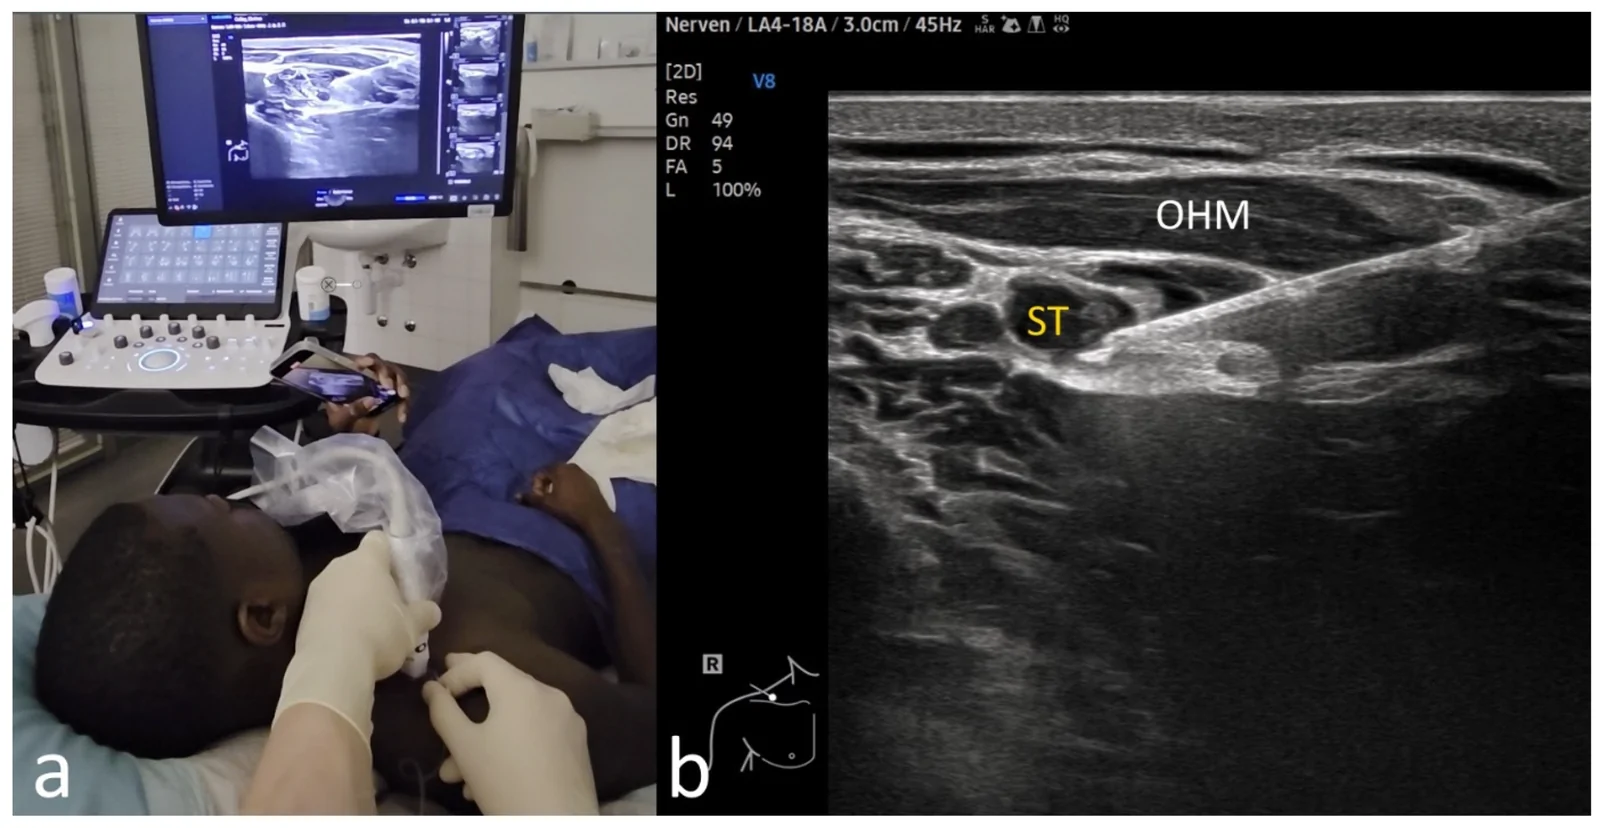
Ultrasound-guided superior trunk block technique. (a) Clinical setup showing the patient position and in-plane needle approach in the supraclavicular region. (b) Corresponding ultrasound image demonstrating the needle tip advanced beneath the superior trunk (ST) with the omohyoid muscle (OHM) as an additional anatomical landmark.
